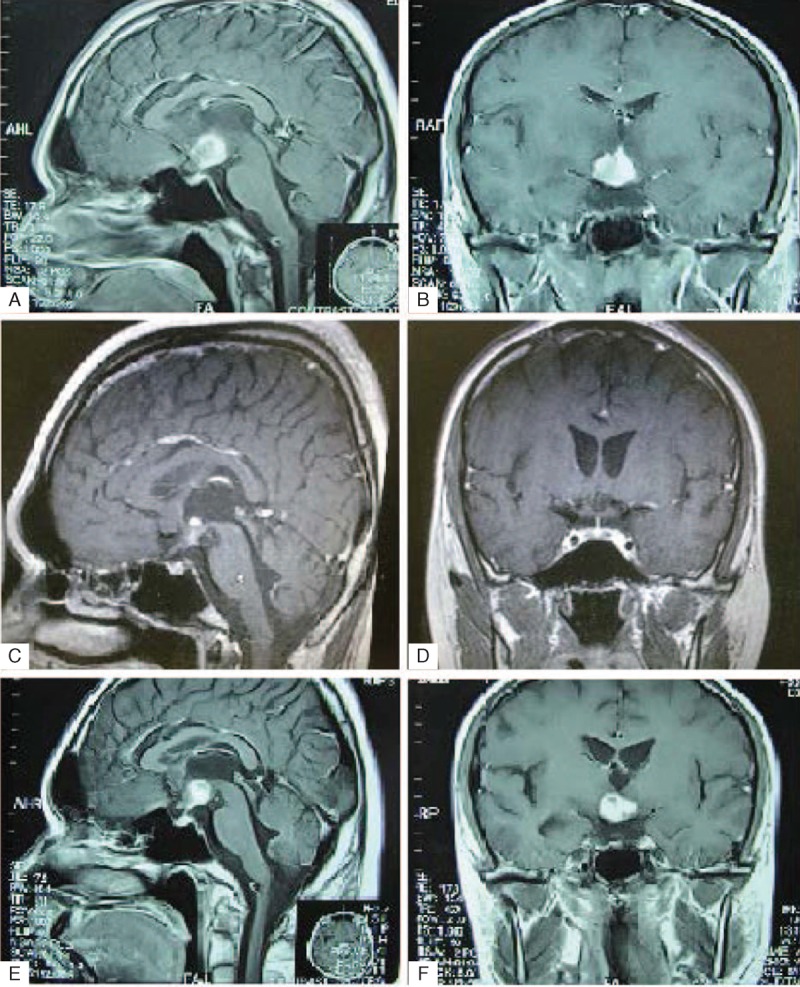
MRI Scans of the patient in Suzhou hospital. Panels A and B show initial imaging, before any treatments. MRI revealed a space-occupying lesion in the hypothalamus. MRI showed decrease in size of the lesion after steroid treatment (Panels C and D). When dexamethasone was gradually withdrawn (to 2.25 mg), the patient's symptoms worsened, and the lesion was seen to enlarge on MRI (Panels E and F). MRI = magnetic resonance imaging.
